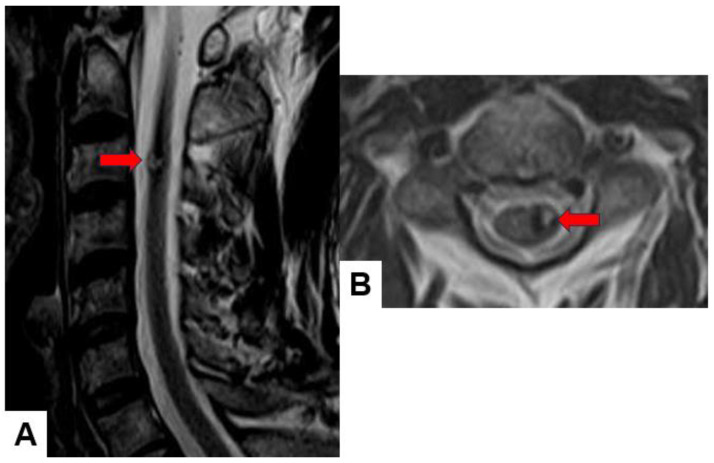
Forty-nine M, cavernous angioma. (A) T2-weighted midsagittal image; (B) T2-weighted axial image at C3. Red arrows indicate tumor.
